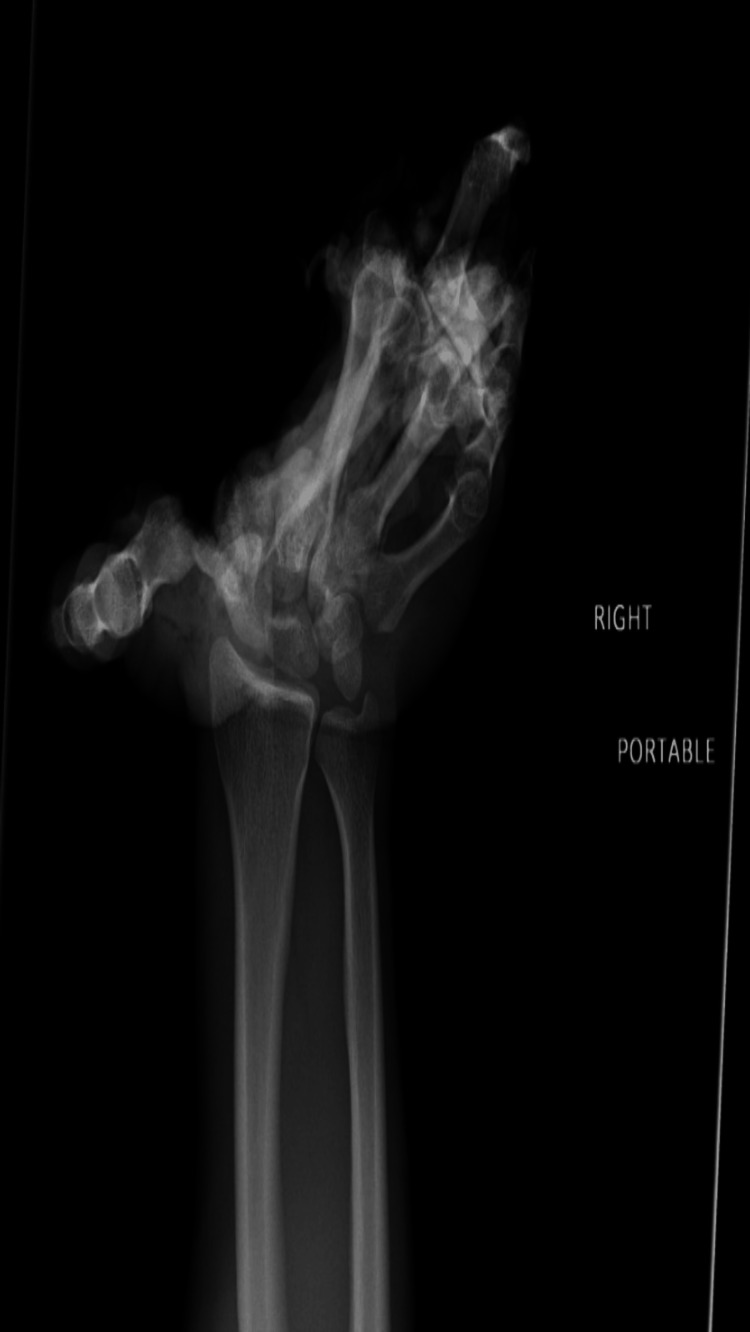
Radiographic image of the right hand on admission. An extreme valgus deformity of the first digit was observed. A dislocation at the base of the first metacarpal was suspected. Disruption of the distal carpal row and its corresponding articulations with the bases of the metacarpals was noted.
